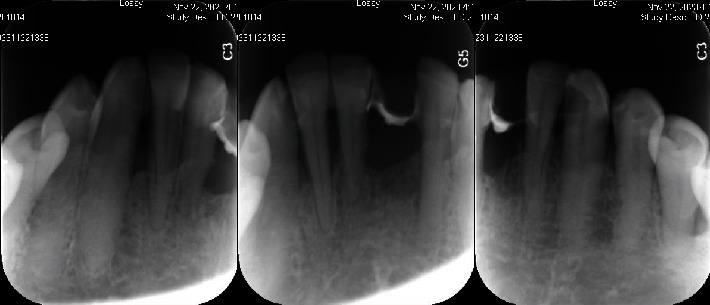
Periapical film follow-up after one-and-a-half years of restoration.
